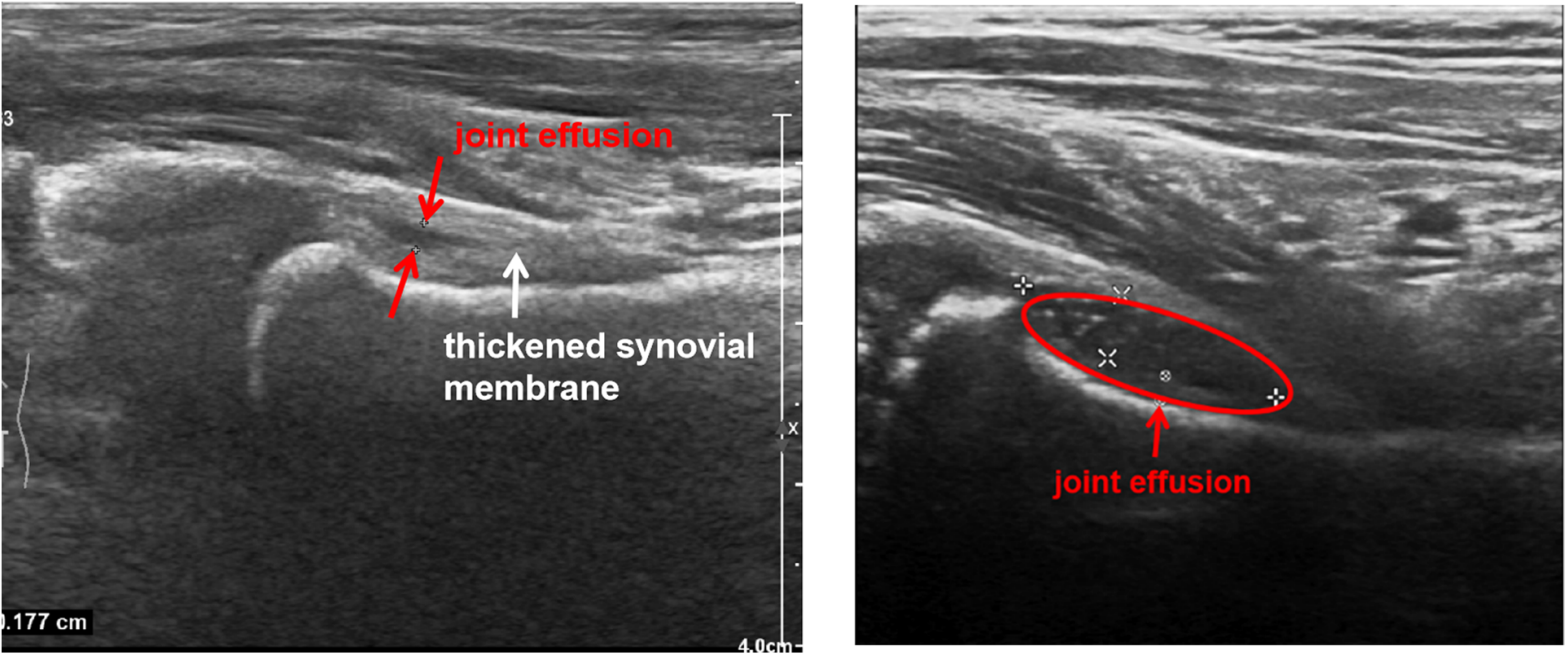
Ultrasound results of kawasaki disease in children with hip synovitis.
